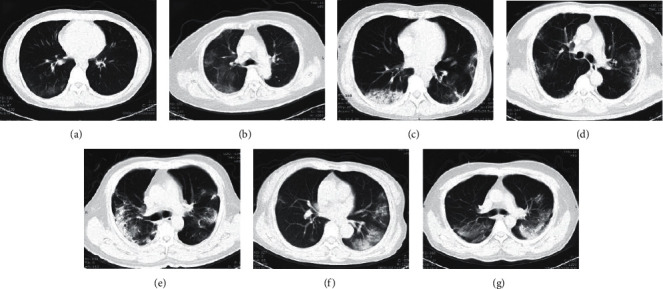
Characteristics of lung CT manifestations in patients with COVID-19.
